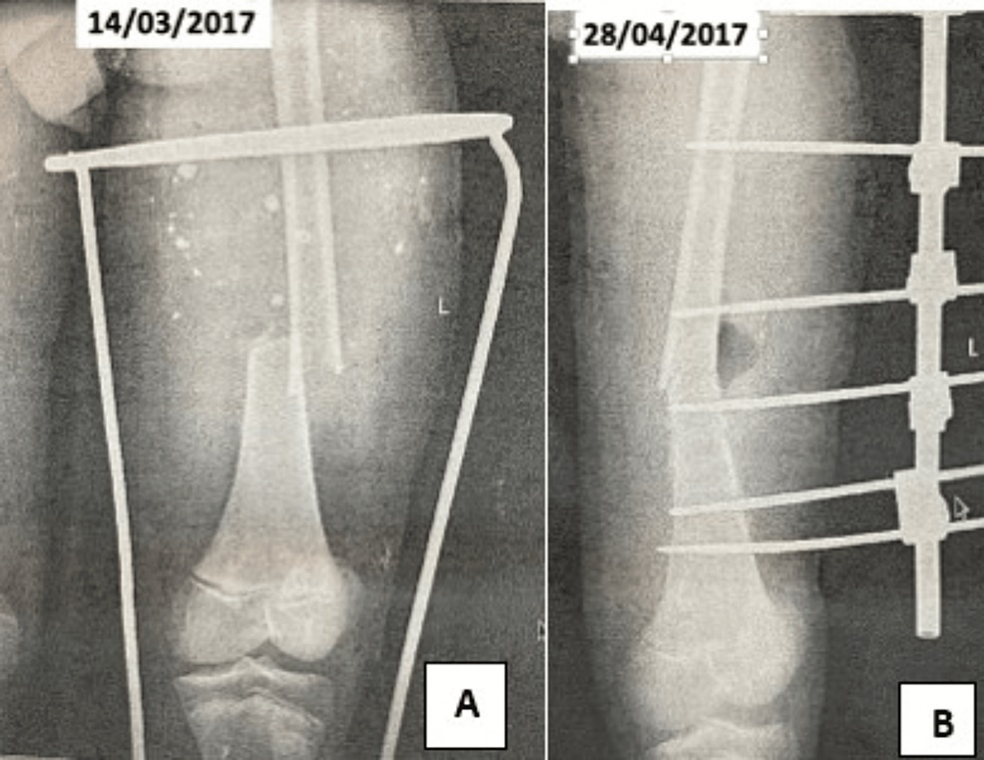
X-ray shows (A) a lower third shaft femur fracture and (B) a unilateral external fixator with angulation at the fracture site.
